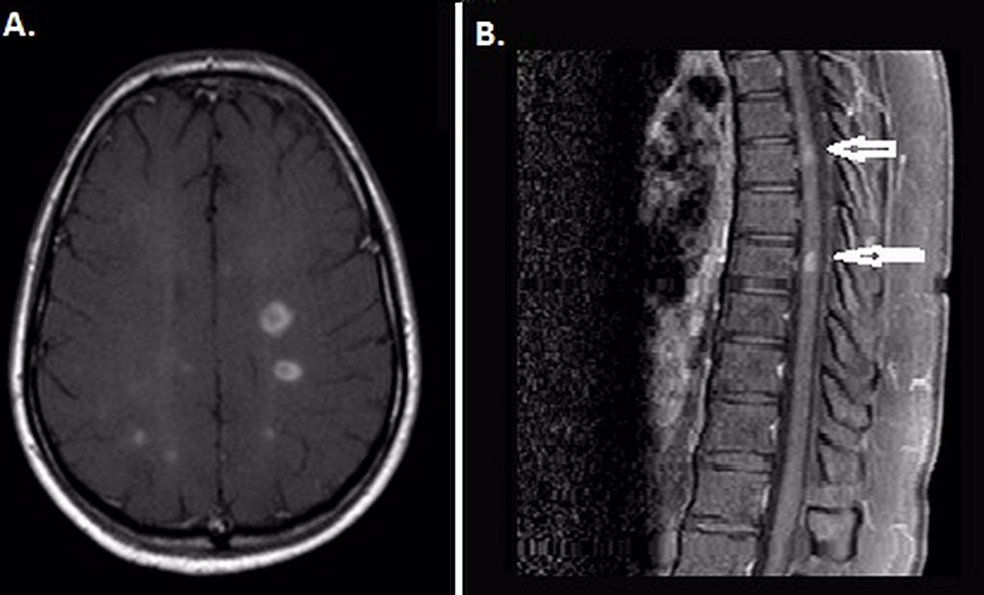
Case 2 five months later MRI After five months, note the development of new contrast-enhancing lesions in the centrum semiovale (A) and thoracal spinal cord (B).
